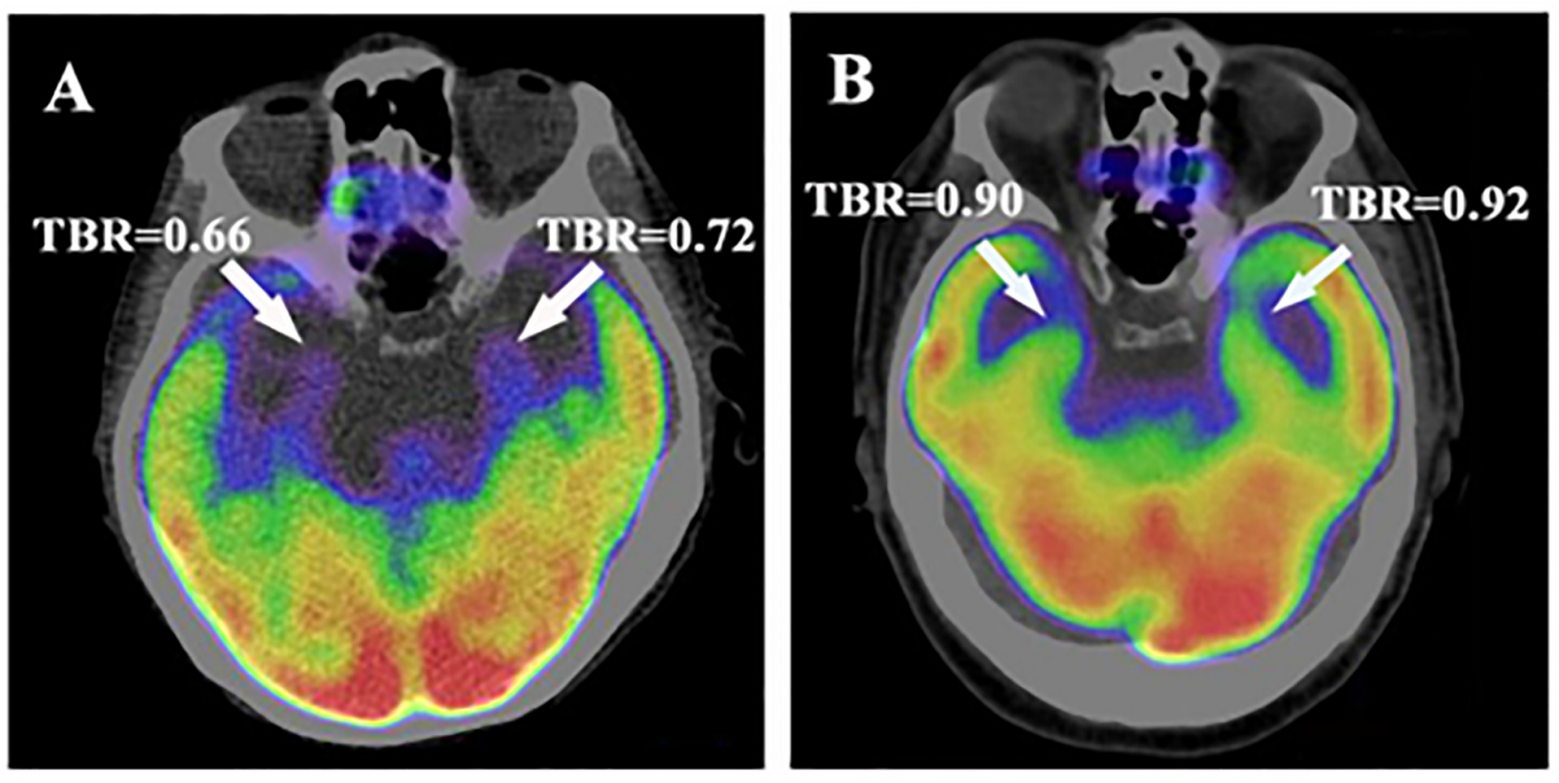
Representative images. Axial views of the amygdala. Higher amygdalar uptake of ^18^F-FDG in a patient who died (A) compared to a patient who survived (B) after a diagnosis of head and neck cancer. These images were recorded at the initial cancer staging prior to cancer treatment. Both individuals had the same stage and type of cancer.
